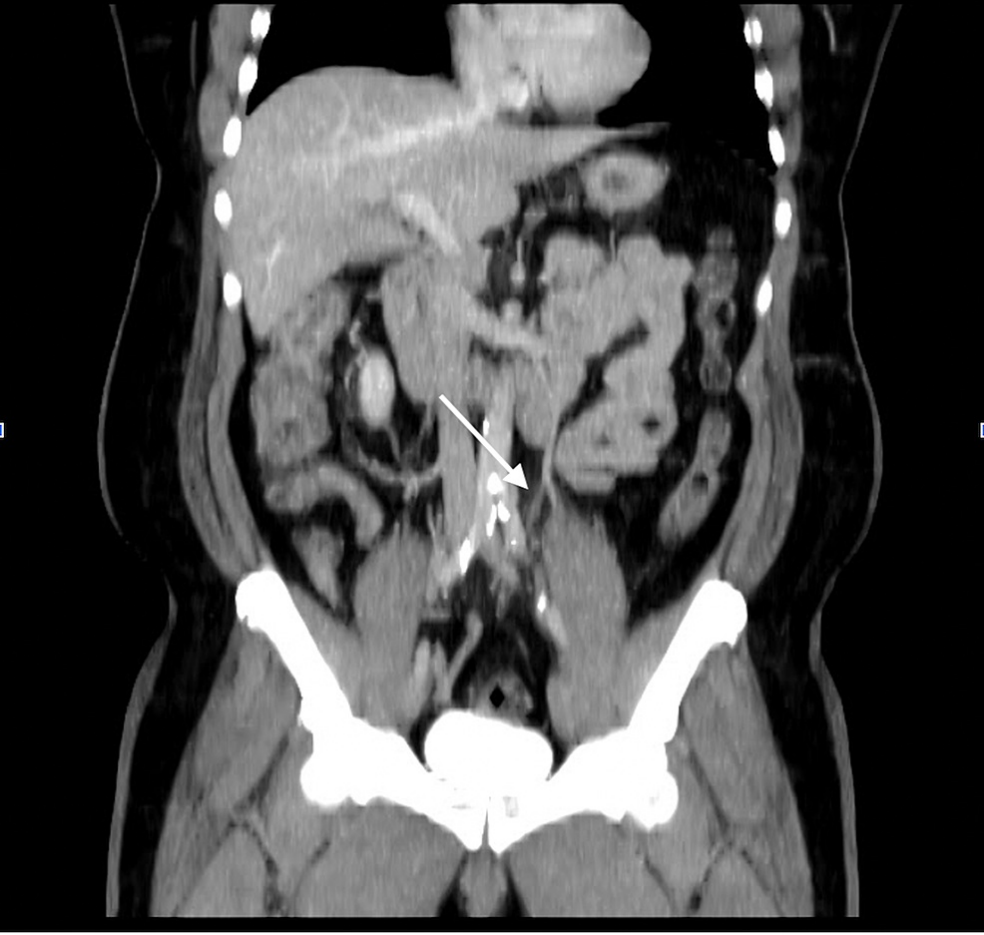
Reformatted coronal view CT abdomen and pelvis with delayed phase showing (arrow) inflammatory changes surrounding the area of partially occluded inferior mesenteric vein. CT, computed tomography.
